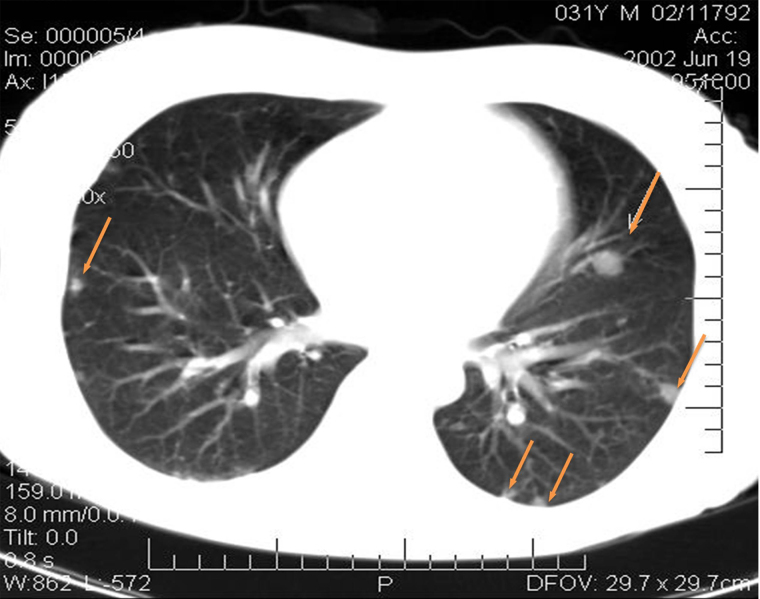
CT images of the patient’s lungs fourteen days after the operation. MTS in the lungs (yellow arrows point at the mts)
